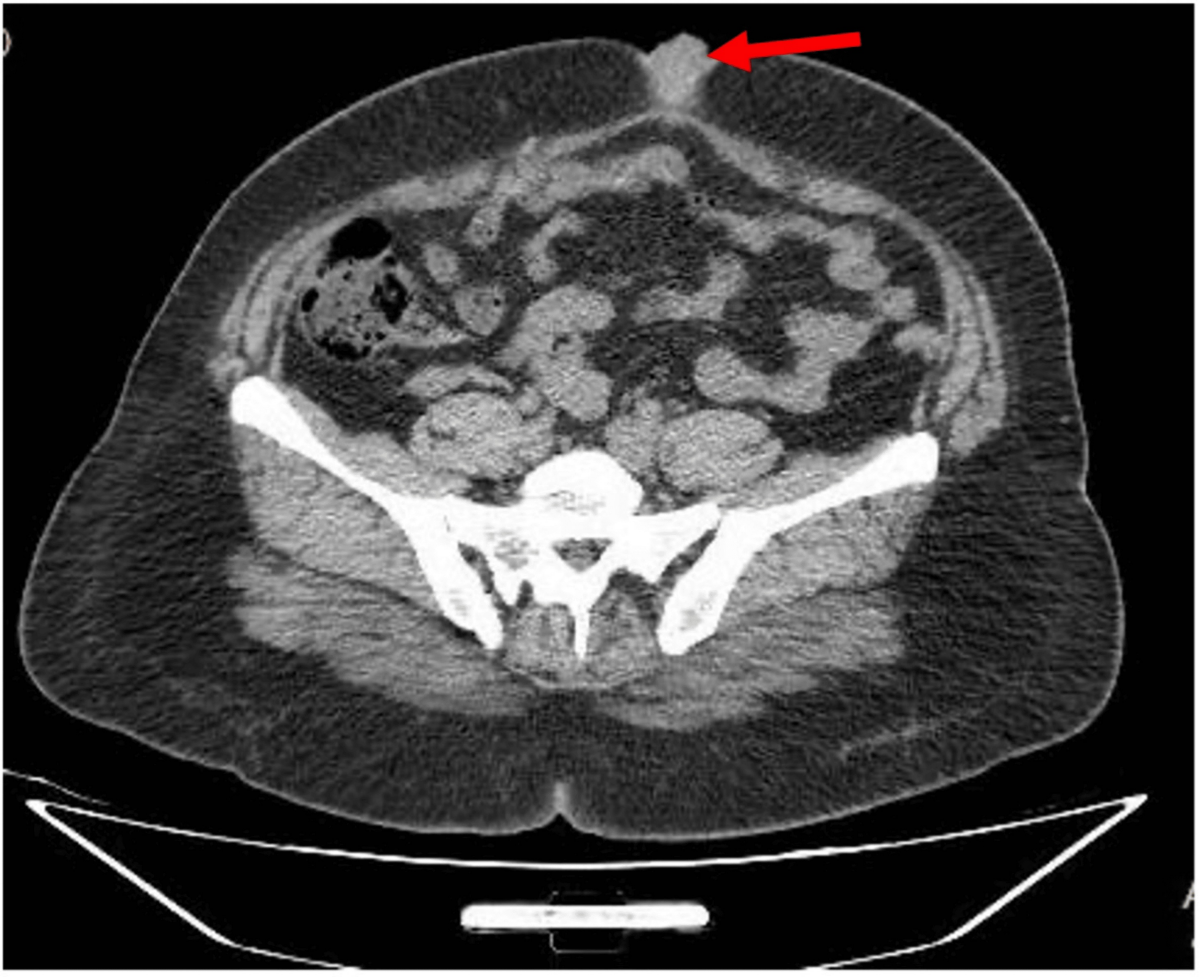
Axial reformatted images of an unenhanced CT scan of the abdomen and pelvis show an irregular soft tissue lesion in the umbilical region measuring 3.0 x 2.6 cm. The lesion is slightly hyperdense in the plain scan with no associated calcifications or cystic changes
